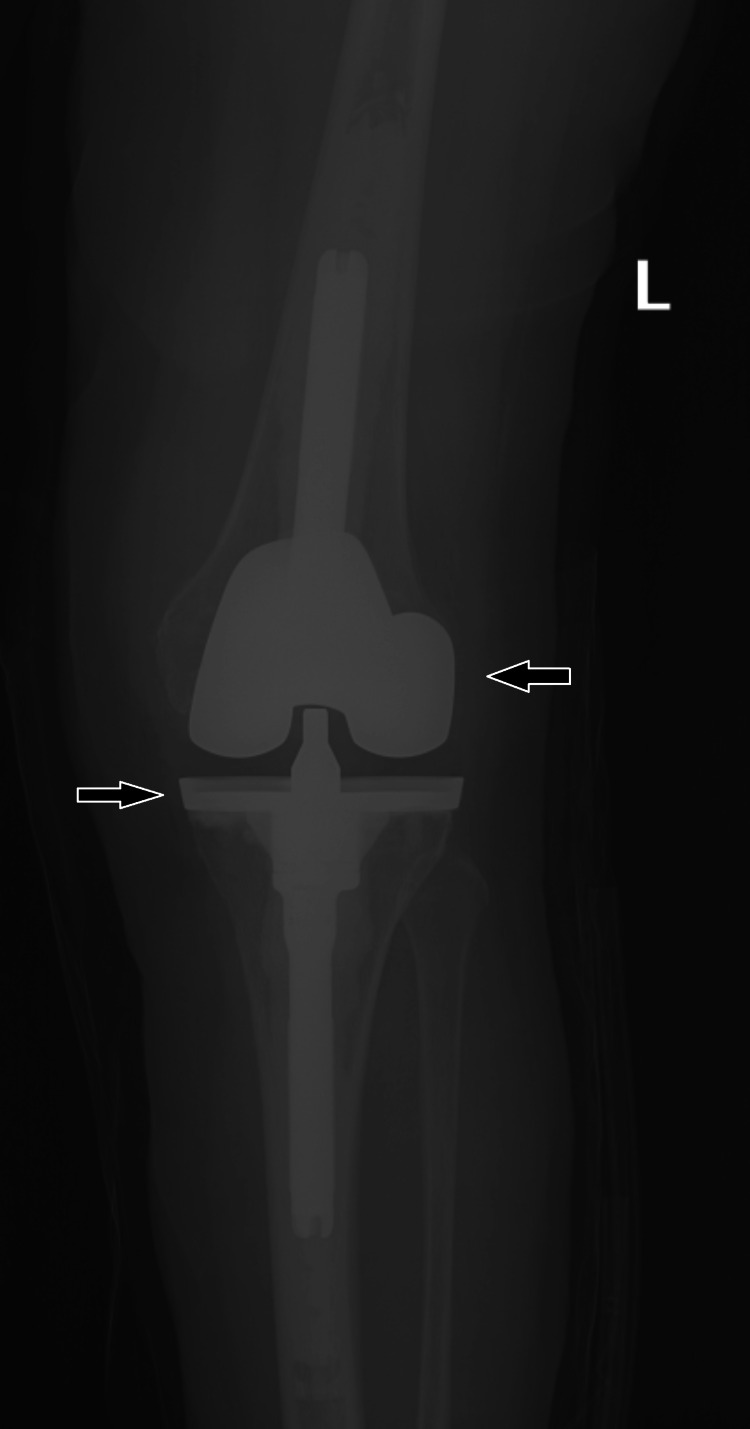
Left knee X-ray showing a DJO Surgical Total Knee Arthroplasty System (black arrows).
